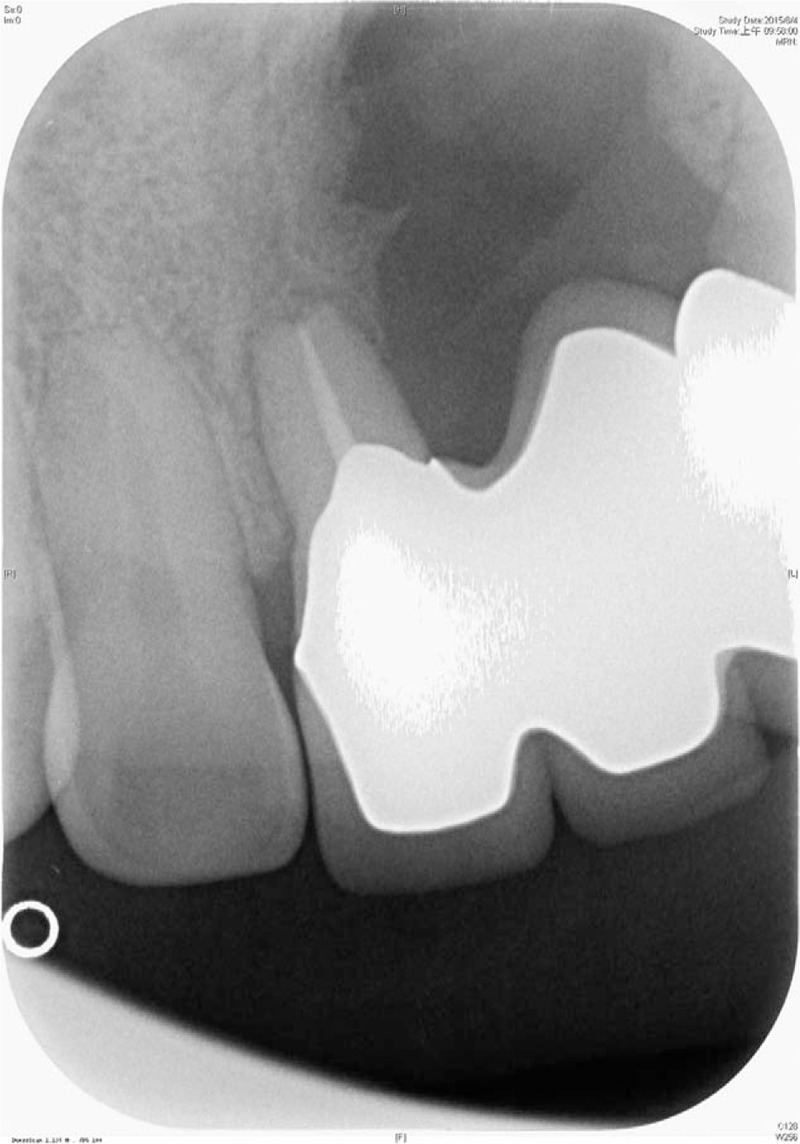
A 31 year-old male patient with unilateral complete cleft lip/palate. He did not have an alveolar bone graft. Radiographs showed peri-dental bone resorption and impending loss of the central incisor. The impact of clinical findings such as these are difficult to assess with questionnaires.
